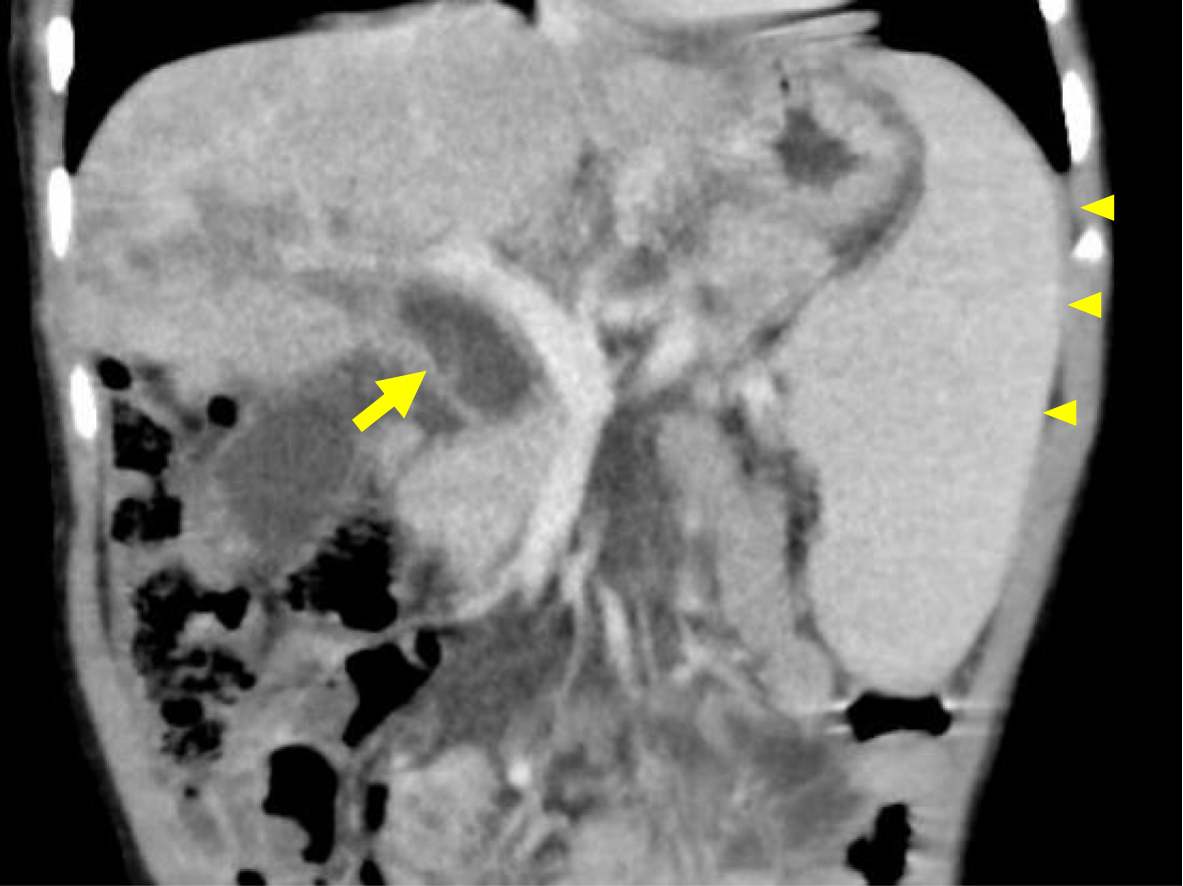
Contrast-enhanced computed tomography of the abdomen (coronal view). The common biliary duct was dilated (arrow). The right hepatic lobe was extremely atrophied, and splenomegaly was recognized (arrow head)
